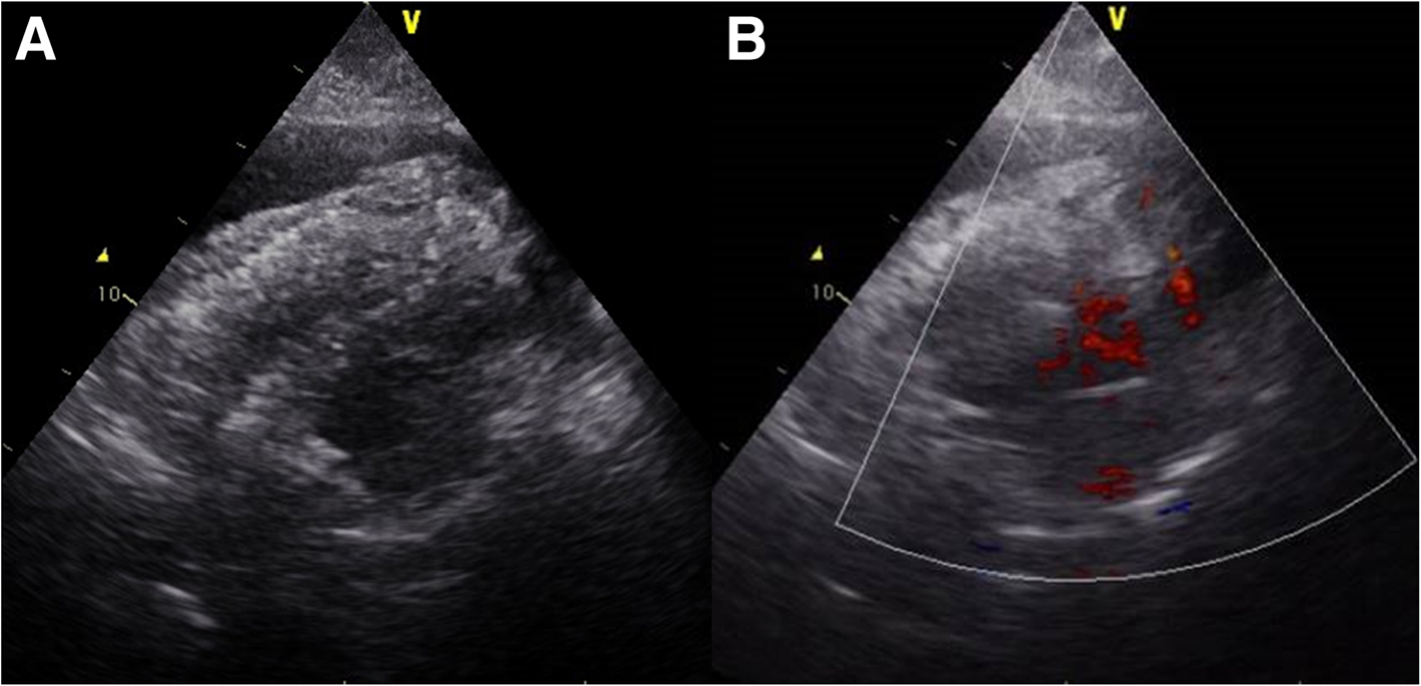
Emergent transthoracic echocardiography. a and b. Intraprocedural transthoracic echocardiography showing the presence of pericardial effusion with hematoma (arrow) and thin left ventricular infero-posterior apical wall (arrowhead)
